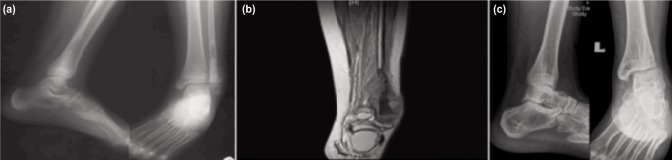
(a) Expansile lytic lesion involving the distal third of fibula with epiphysis with some patchy sclerosis and cortical breach, (b) MRI of left fibula showing mass lesion within and encircling the distal fibula with proximal extension of the marrow and cortex with central necrosis and (c) Post-surgical follow up; Radiograph of ankle joint shows valgus and talar shift with no signs of degeneration in last follow up in 20 December 2016.
